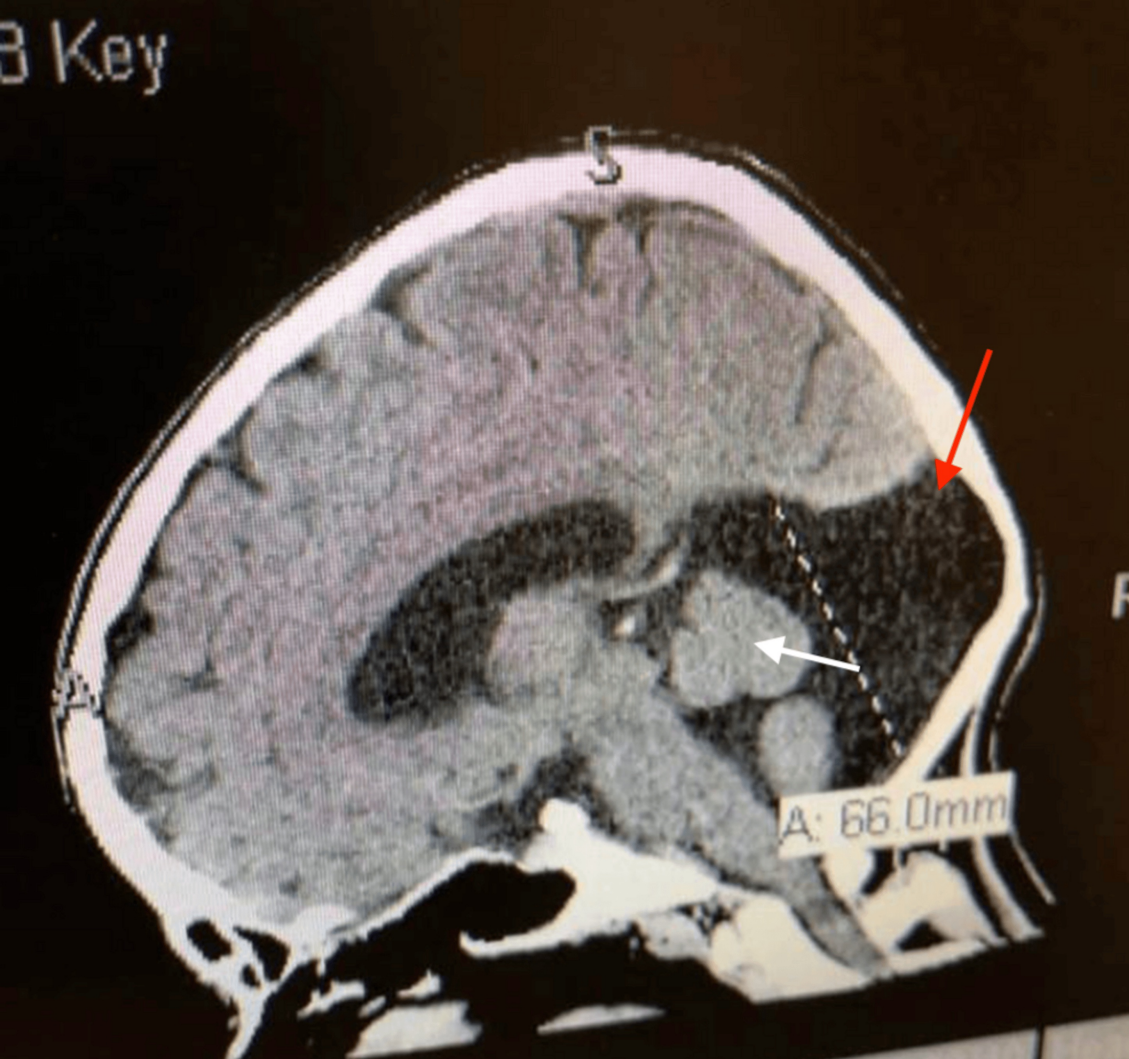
Sagittal non-contrast CT of the patient's brain demonstrating hypoplasia of the cerebellar vermis and cystic enlargement of the posterior fossa (arrows), consistent with Dandy-Walker malformation Scale bar=66 mm (11 divisions at 6 mm each) White arrow: cerebellar vermis hypoplasia; red arrow: cystic enlargement of the posterior fossa CT: computed tomography
